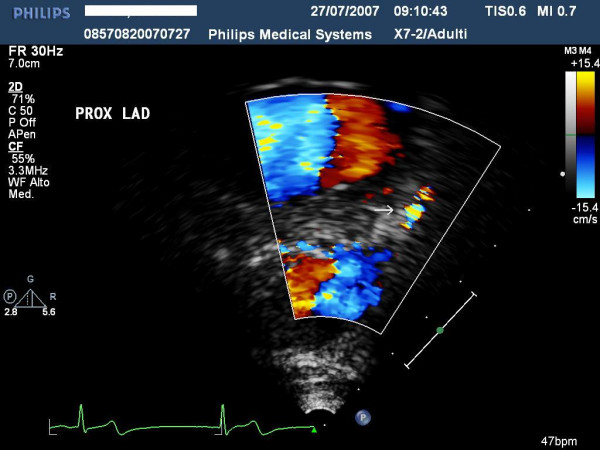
highlights the ultrasound findings of proximal left anterior highlighted with color-Doppler and.
